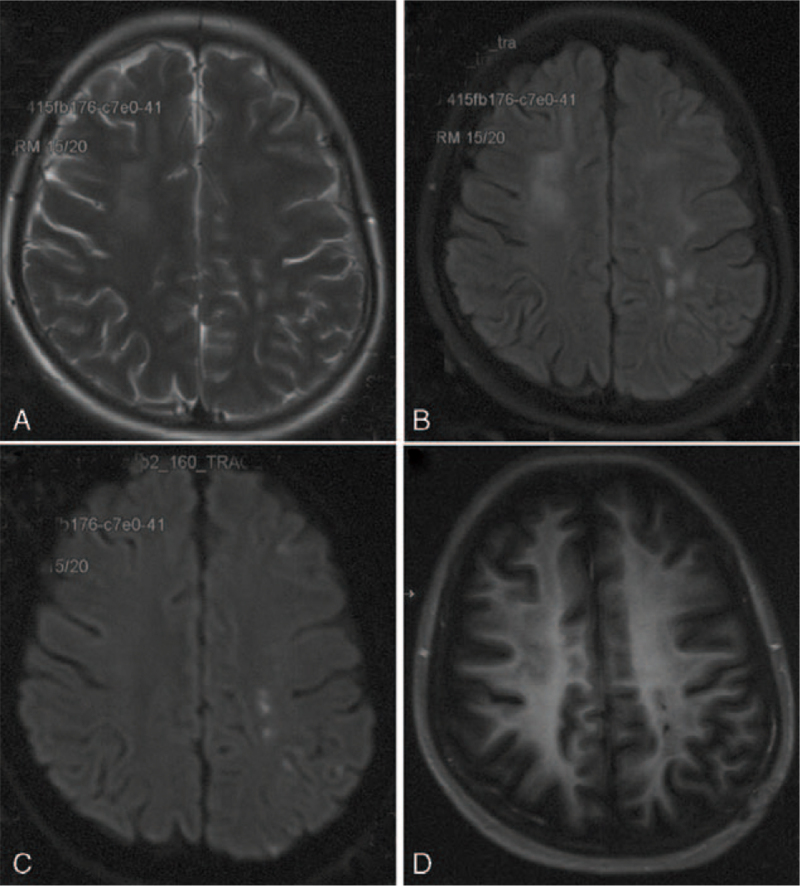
Brain MRI before admitted revealed bilateral fronto-parietal subcortical white matter abnormal signal with slightly higher T2WI and FLAIR signal and hyperintense of left fronto-parietal lobe on DWI (A–C). No enhancement was found on brain enhanced MRI after admission (D). FLAIR = fluid attenuated inversion recovery, DWI = diffusion weighted imaging; MRI = magnetic resonance imaging, T2WI = T2 weighted image.
